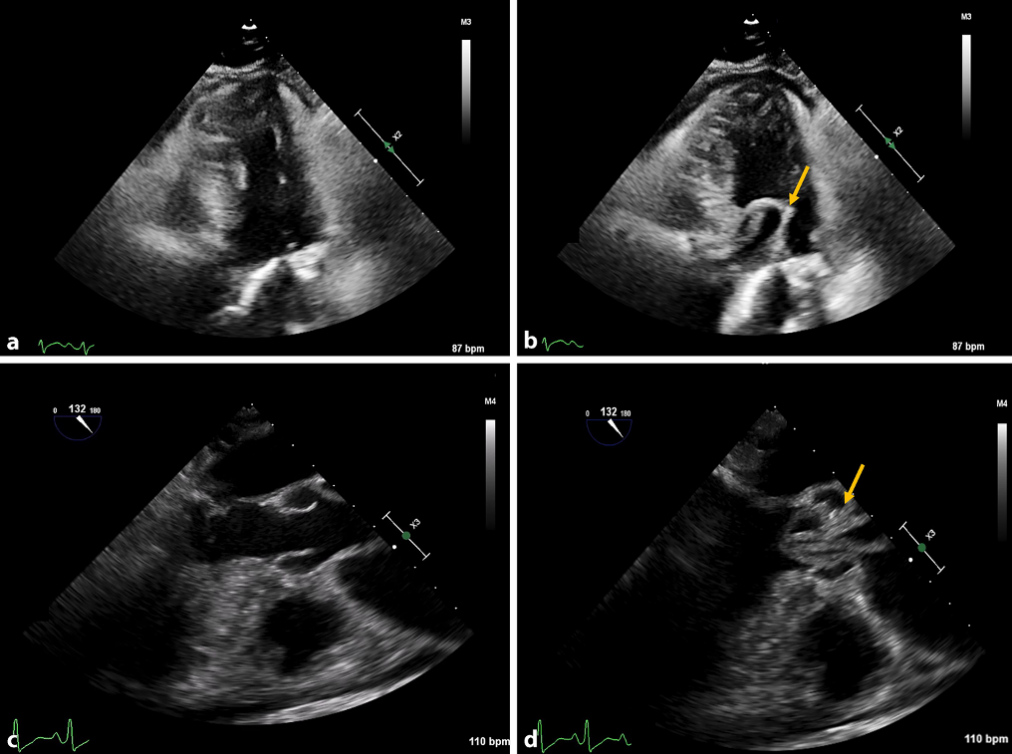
Echocardiography images of intimointimal intussusception. a Transthoracic echocardiography five-chamber view, systolic phase. b Transthoracic echocardiography five-chamber view, diastolic phase. c Transesophageal echocardiography 132°, systolic phase. d Transesophageal echocardiography 132°, diastolic phase. Yellow arrow indicates the intimointimal intussusception
